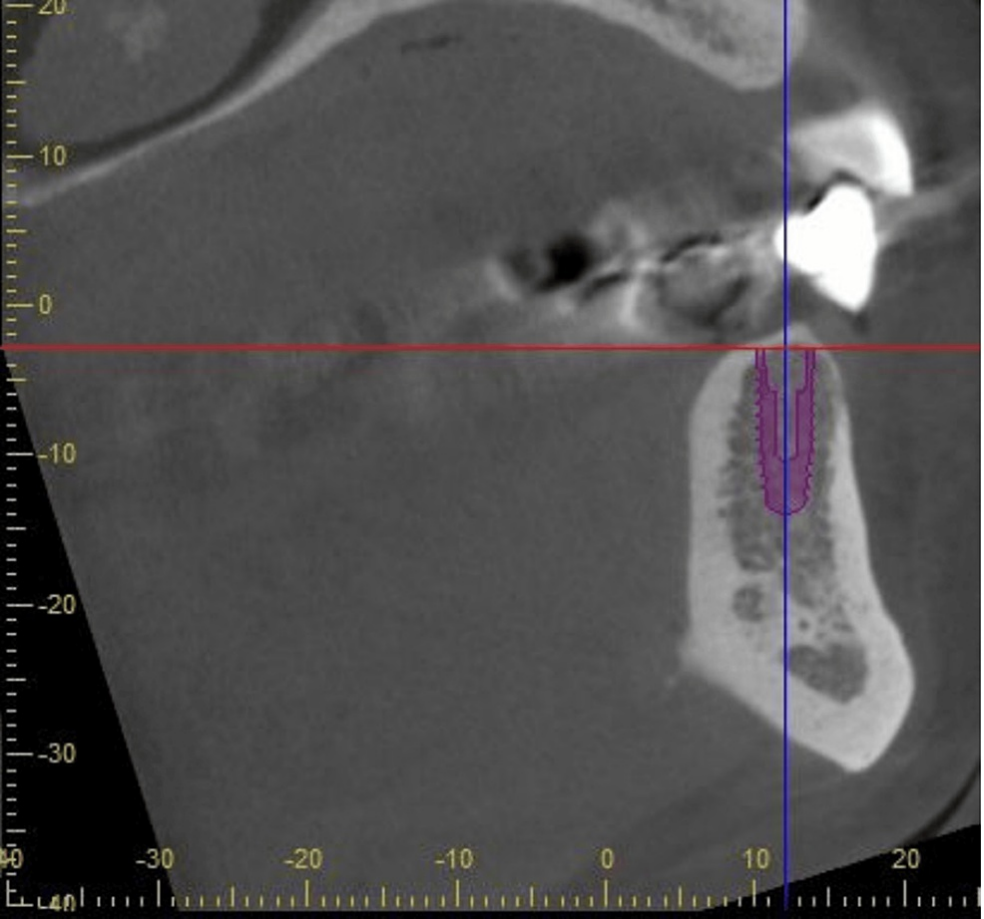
Sagittal view of pre-operative CBCT demonstrating implant planning CBCT: cone-beam computed tomography
